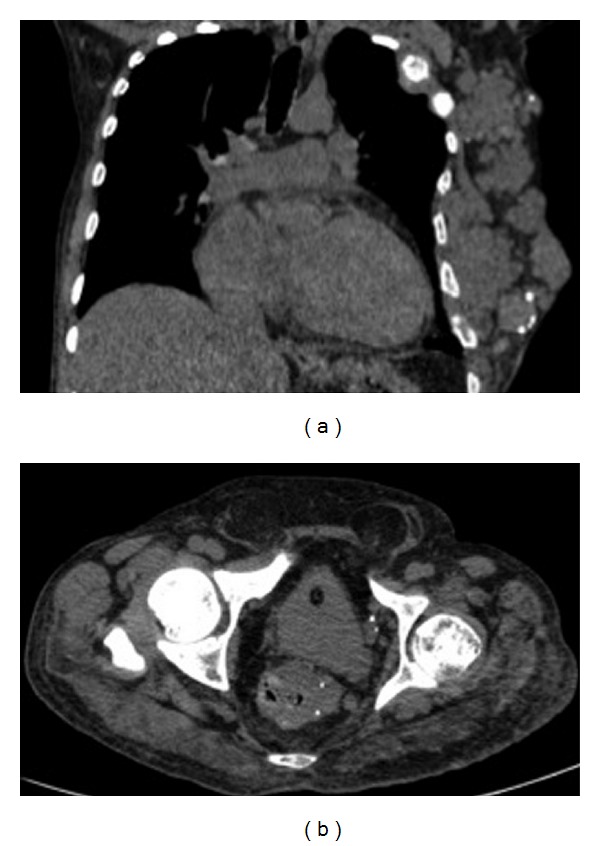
A 60-year-old man with Klippel-Trenaunay-Weber syndrome presenting asymmetric growth of the lower limbs. CT of the chest showed increased soft tissue as well as extensive vascular malformations in the left hemithorax wall with intermingled phleboliths, causing multiple lytic lesions with enlargement in the ipsilateral ribs (a). CT of the abdomen showed a thick-walled rectum intermingled with phleboliths, denoting varicose veins (b).
